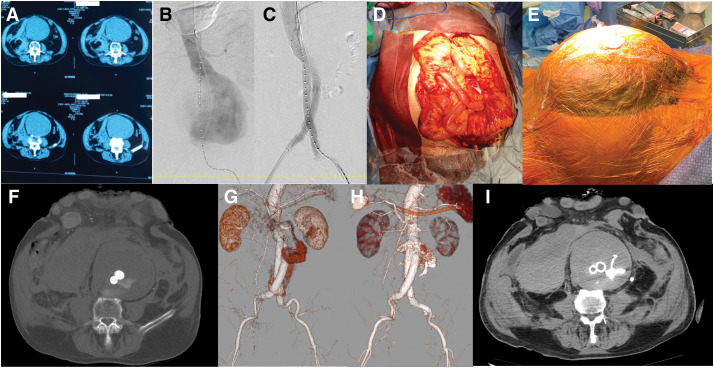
Timeline of surgical interventions. Preoperative plain CT imaging (A). DSA showed preoperative AAA (B). DSA after EVAR (C). Bowel findings during open abdominal decompression (D). Abdominal findings with Abthera and open management (E). Enhanced CT findings about type-1b endoleak after EVAR (F and G). Enhanced CT findings after placing iliac extender and infusion of N-butyl-2-cyanoacrylate (H). Retroperitoneum large hematoma and giant AAA after EVAR and additional procedure (I). AAA, abdominal aortic aneurysm; EVAR, endovascular aortic repair
